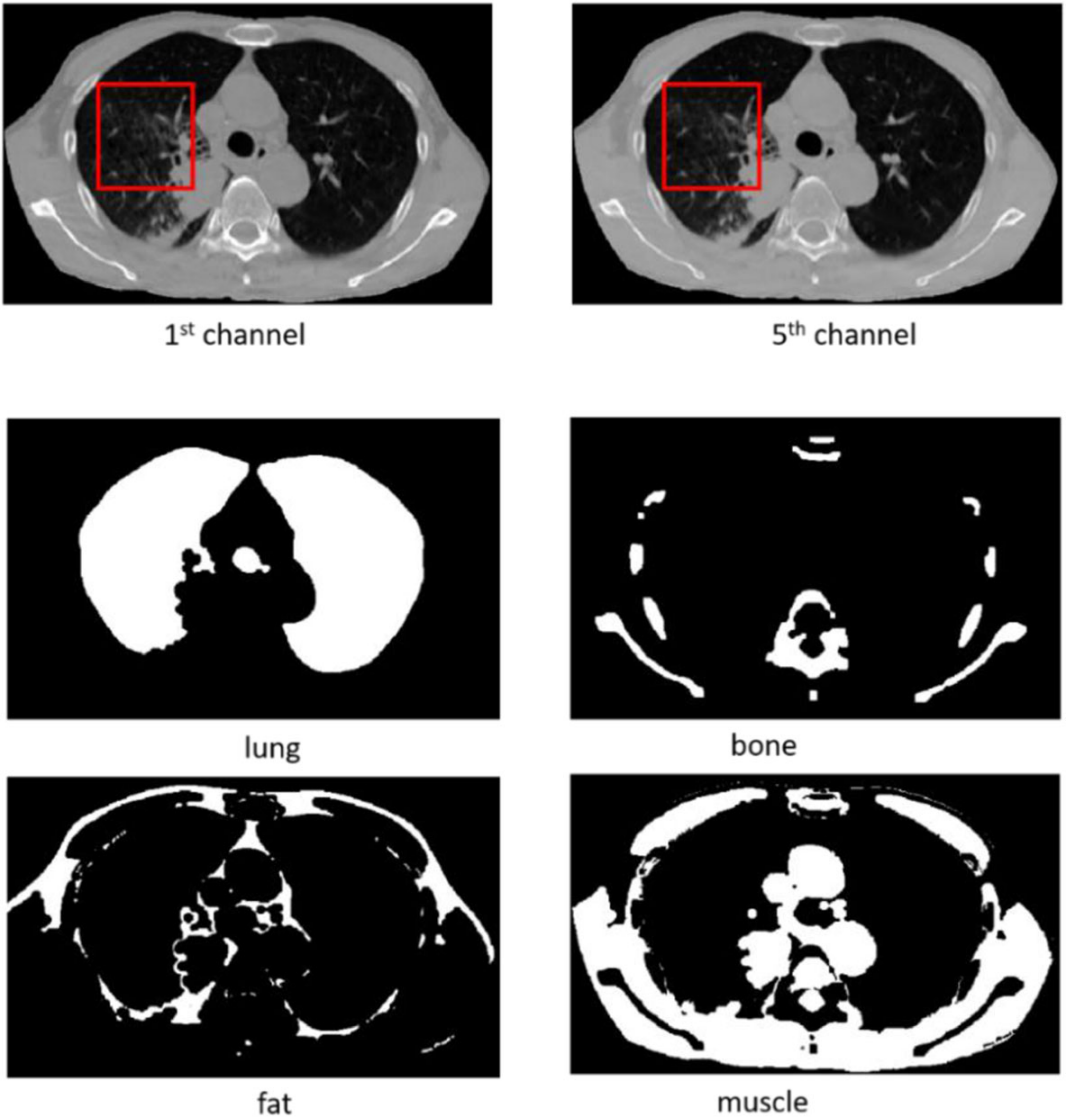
Full dose computed tomography images and their segmented tissue masks. Images of first and last (5th) energy channel. Tissue masks of lung, bone, fat and muscle
